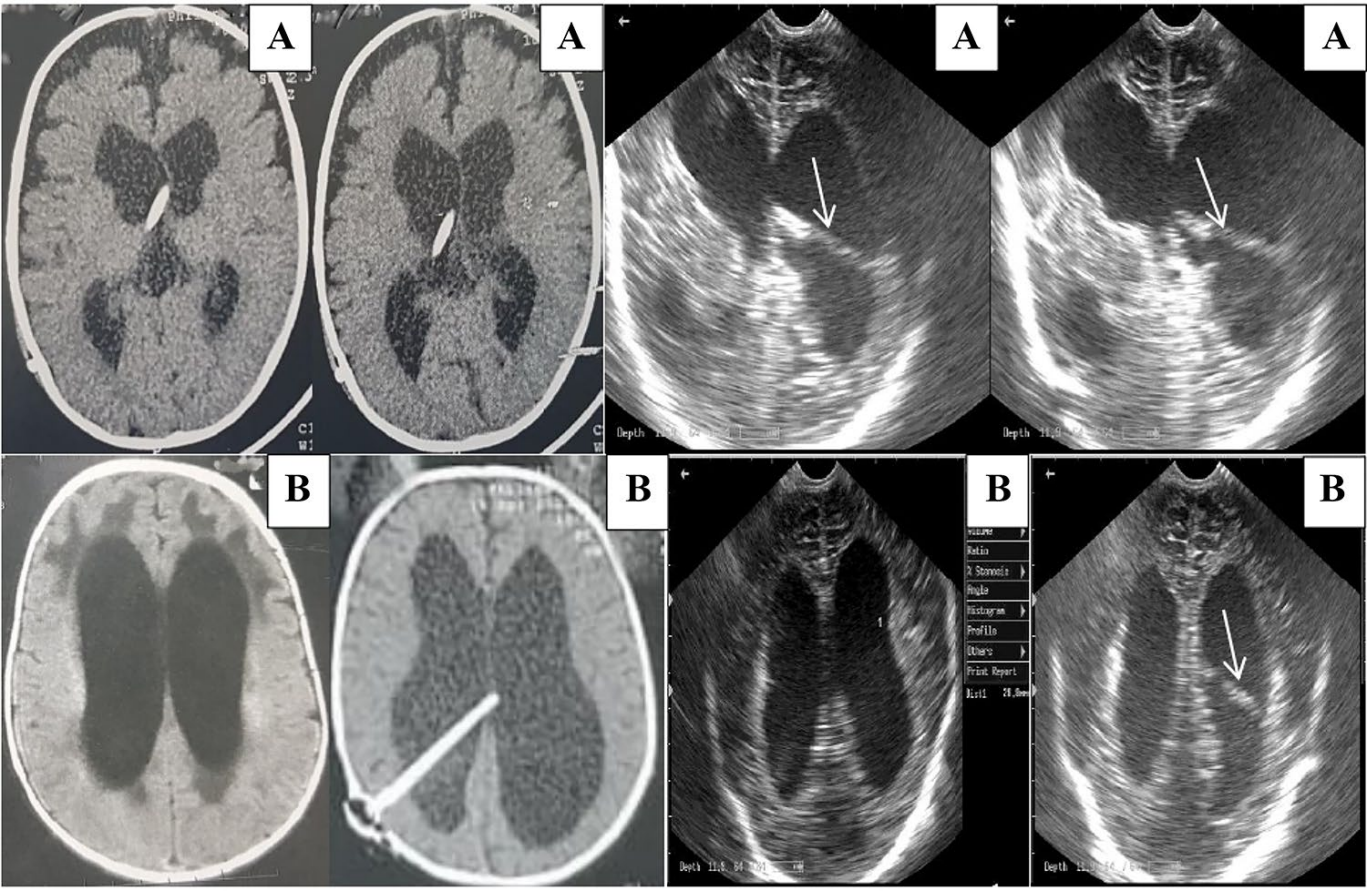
Equivalent CT and ultrasound images. Case A, with a catheter inside the frontal horn of the lateral ventricle. Case B, CT and ultrasound images before and after catheter insertion inside the body of the lateral ventricle. Thin arrows refer to the catheter inside the ventricles
